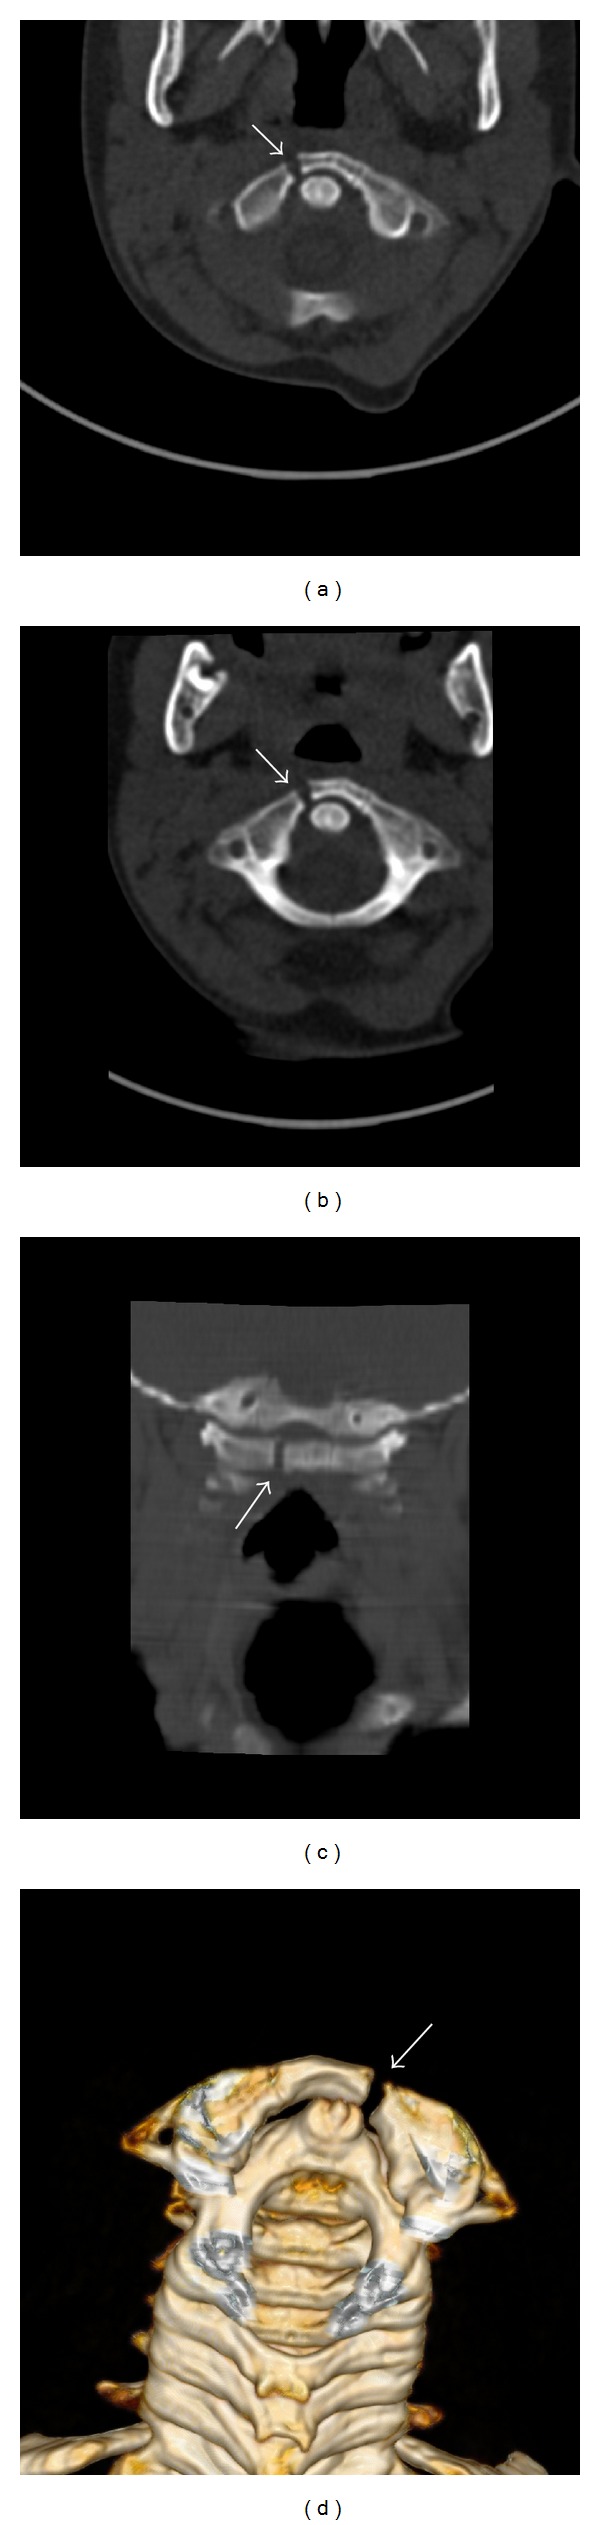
Axial plan, CT scan of cervical vertebrae with 2 mm slice thickness (a), axial oblique (b), coronal oblique (c), MIP reconstruction, and posterior view of 3D volume rendering (d) images show fracture of the atlas through a synchondrosis of the anterior arch (arrows). The space at right synchondrosis line is measured 3.5 mm.
